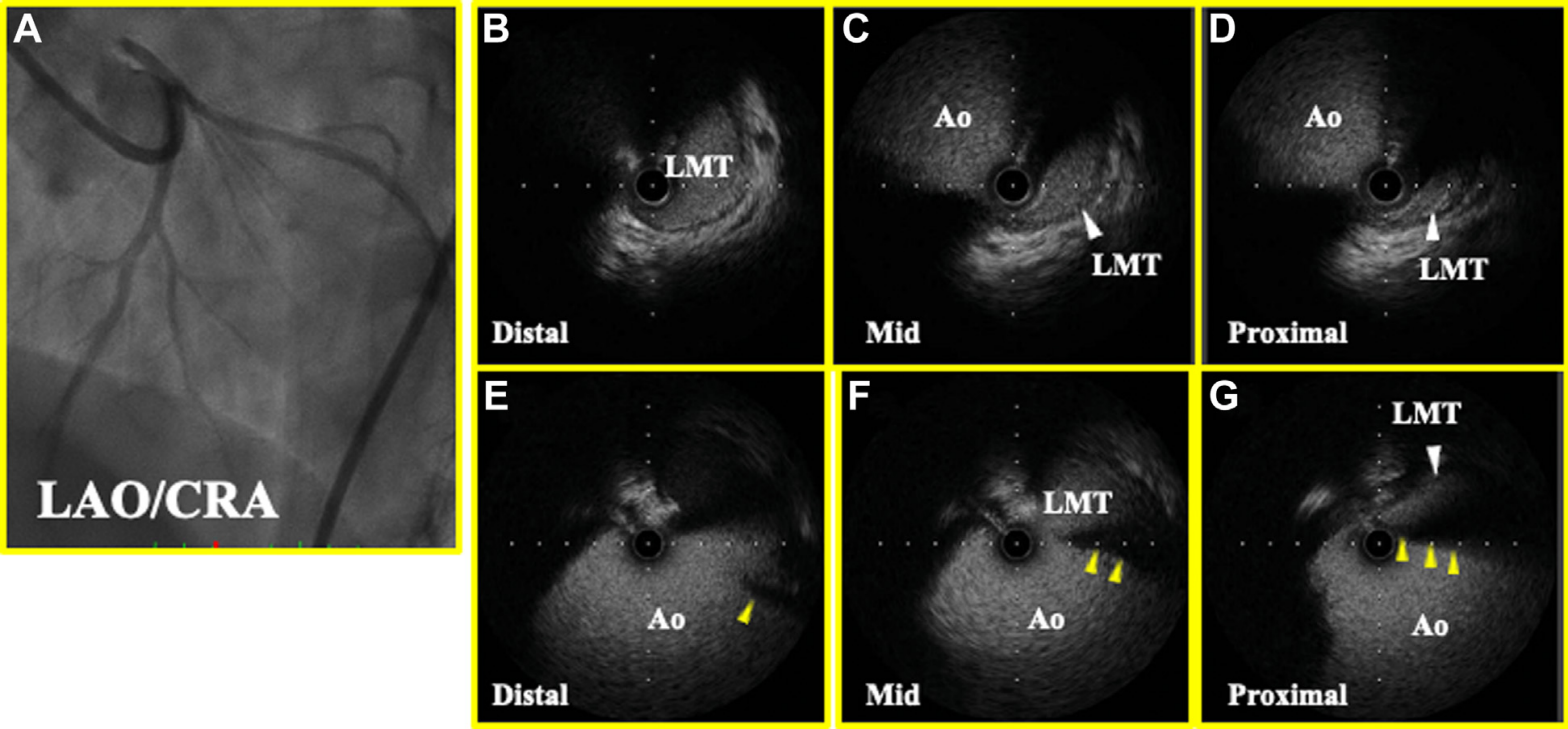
Enhanced Angiogram and IVUS (A) Enhanced angiogram from LCC of LAD, showing slit-like appearance in ostium of LMT (yellow arrowhead). (B to D) IVUS from LMT showing black shadowing, indicating valve-like ridge (yellow arrowhead). (E to G) IVUS from ascending aorta. Ao = aorta; CRA = cranial position; IVUS = intravascular ultrasound; LAD = left anterior descending artery; LAO = left anterior oblique position; LCC = left coronary cusp; LMT = left main trunk.
